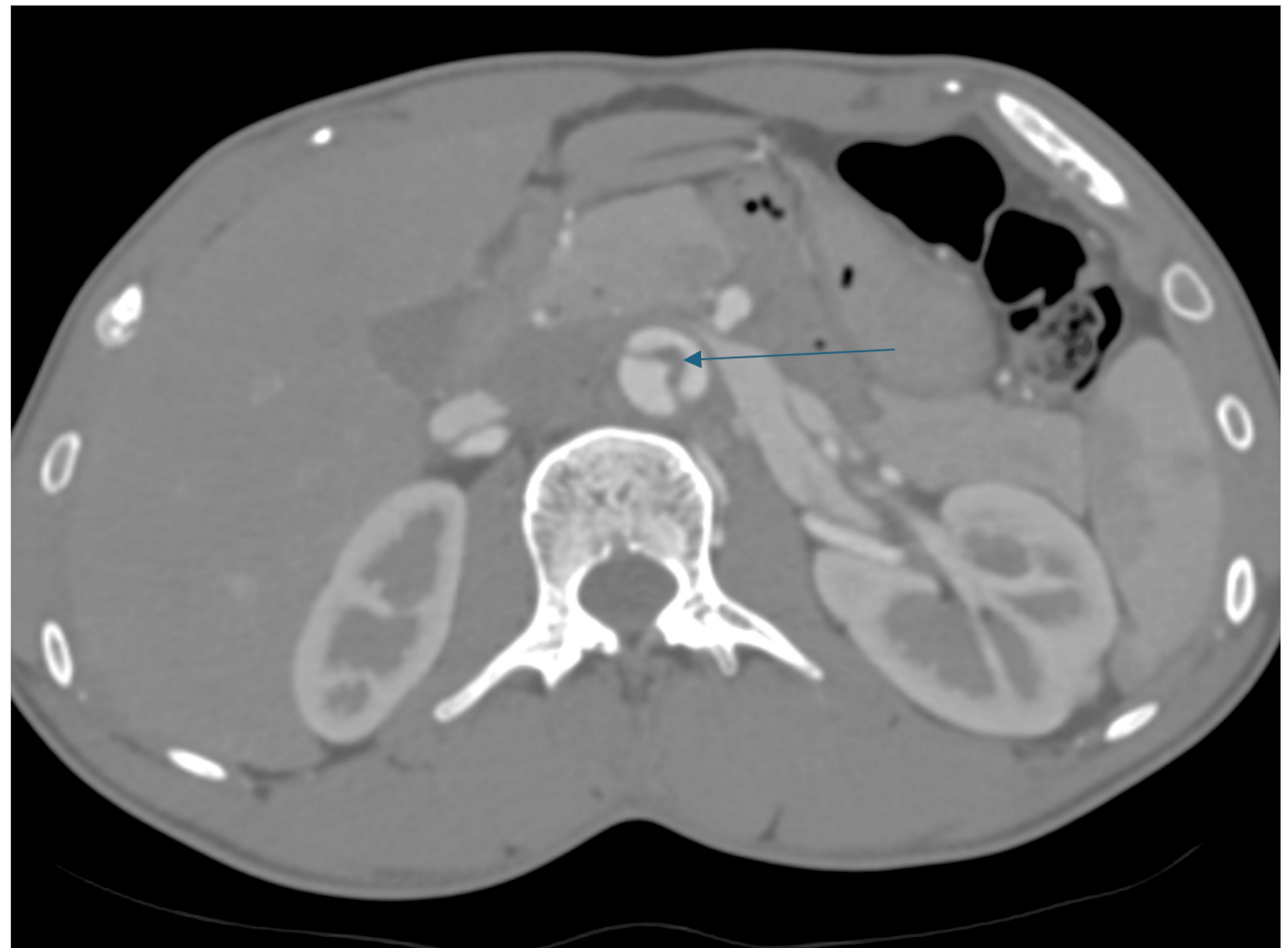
CECT abdomen axial section showing hypodense dissection flap (shown by thin arrow) within the lumen of the abdominal aorta CECT: contrast-enhanced computed tomography
